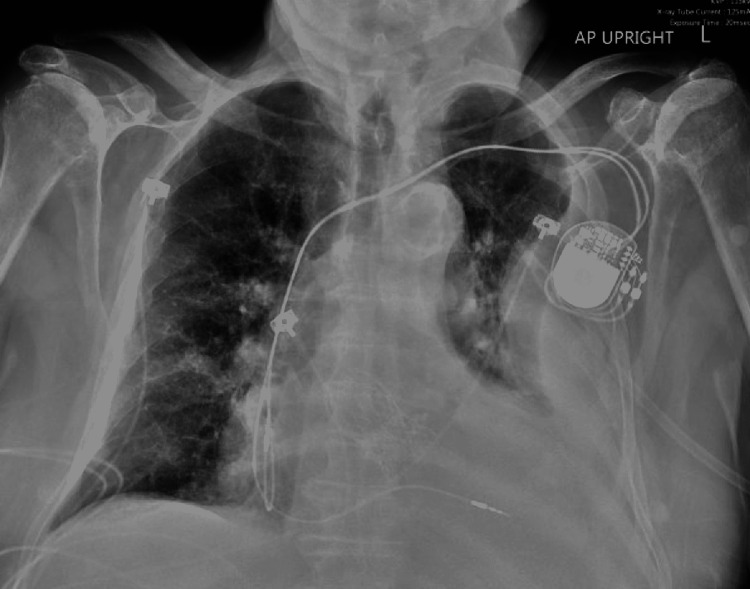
Chest X-ray anteroposterior view demonstrating left pleural effusion
